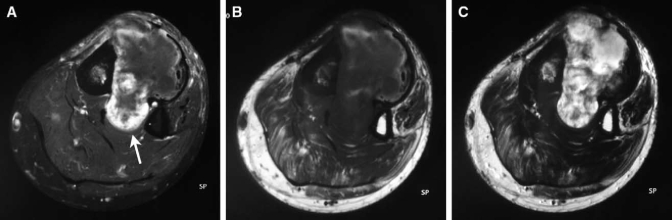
Magnetic resonance imaging demonstrated that the contents of the masses were isointense with muscles on T1-weighted images and hyperintense on T2-weighted images, with peripheral low signal intensity foci representing calcification. Postcontrast fat-suppressed T1-weighted image showed peripheral ring enhancement (arrow).
